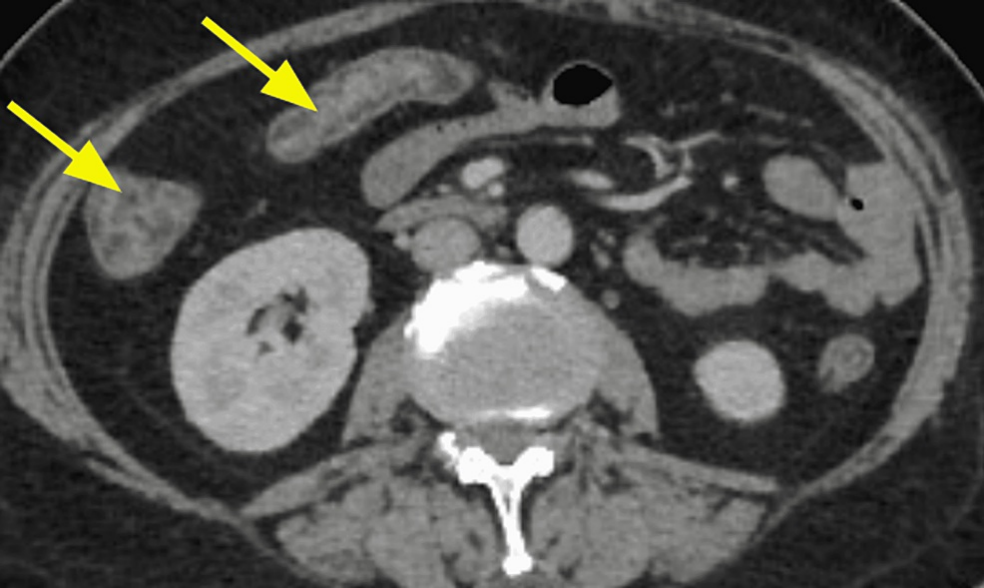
Contrast-enhanced CT image for Case 4 (axial plane) demonstrating thickening of the large bowel wall and increased mucosal enhancement (arrows)
